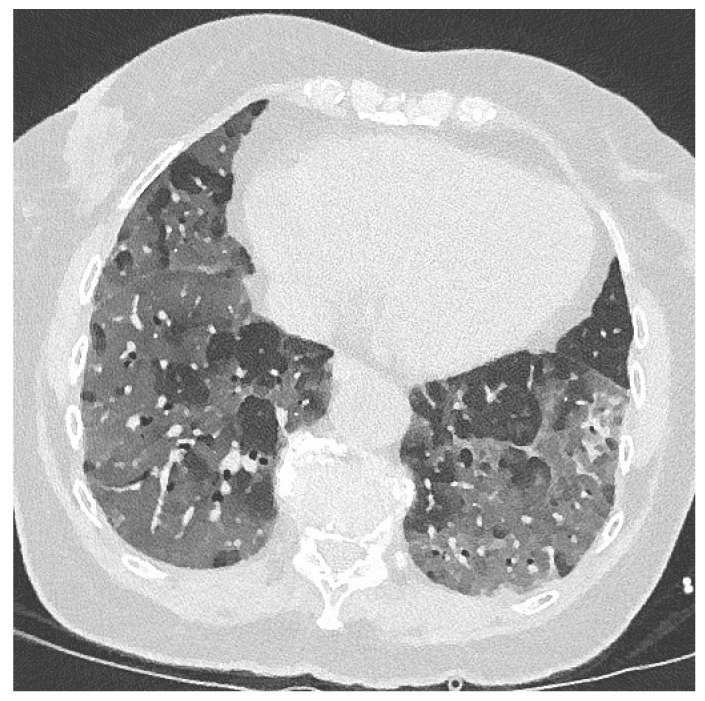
Low-dose chest CT showing extensive centralized and peripheral bilateral ground glass opacities with left-sided consolidations and bilateral fibrotic bands. No pleural effusion. No vascular enlargement and no specific suggestions of aspergillosis.
